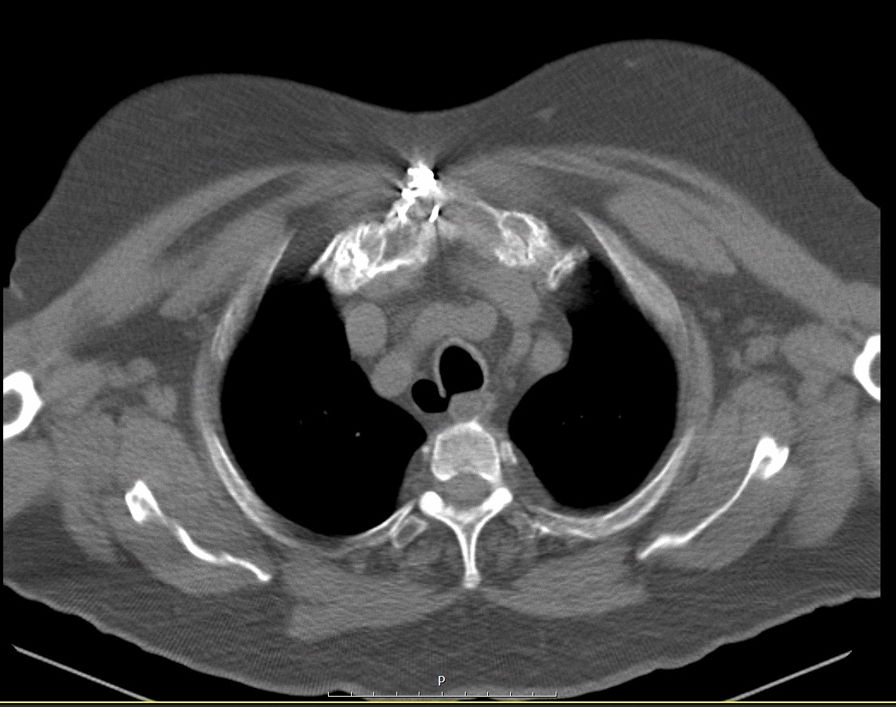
Axial bone window of chest computed tomography (CT) scan
